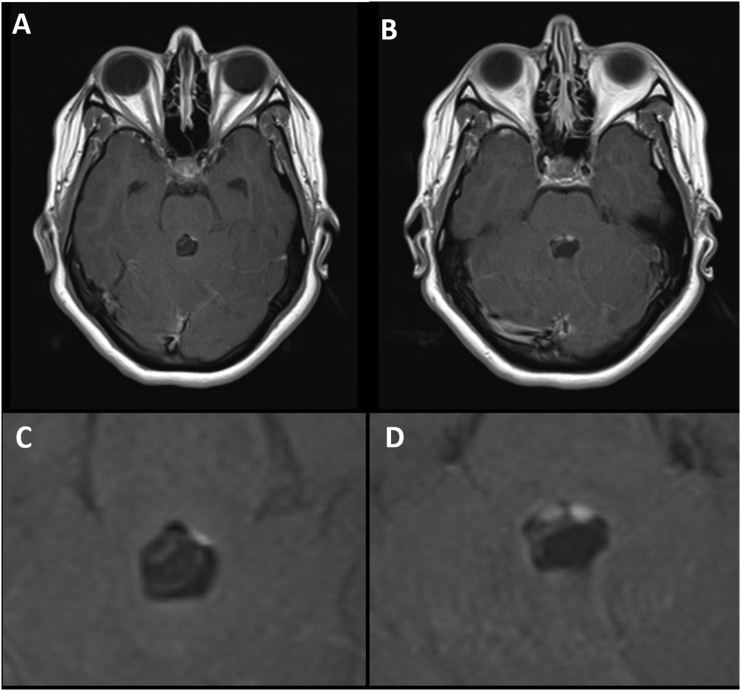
Higher magnification of preoperative Magnetic Resonance Imaging (MRI) axial scans showing racemose neurocysticercosis (NCC) lesion (A, C) pre-contrast, and (B, D) post-contrast.
